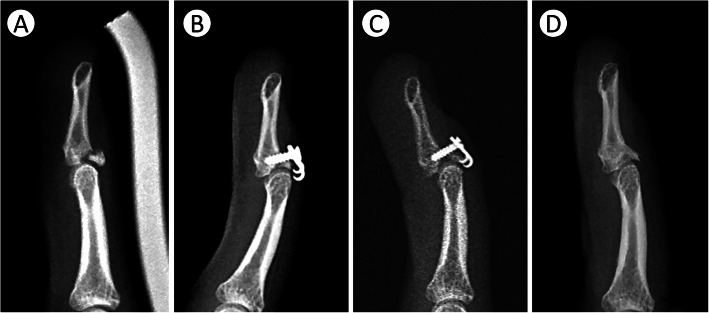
Suboptimal plate and screw placement. a The pre-operative radiograph showed mallet fracture without DIP joint subluxation. b The post-operative radiograph showed that the screw was inserted through the fracture site and failed to purchase the far cortex. c Implant loosening with fracture fragment displacement was found at 1-month follow-up. d The hook plate was removed after bone union
